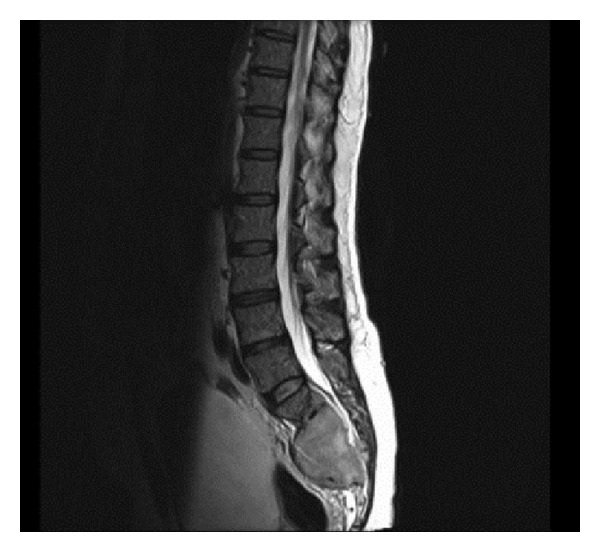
MRI revealing large soft tissue mass in sacral region causing severe canal stenosis and compression of the sacral nerve roots resulting in cauda equina syndrome.
